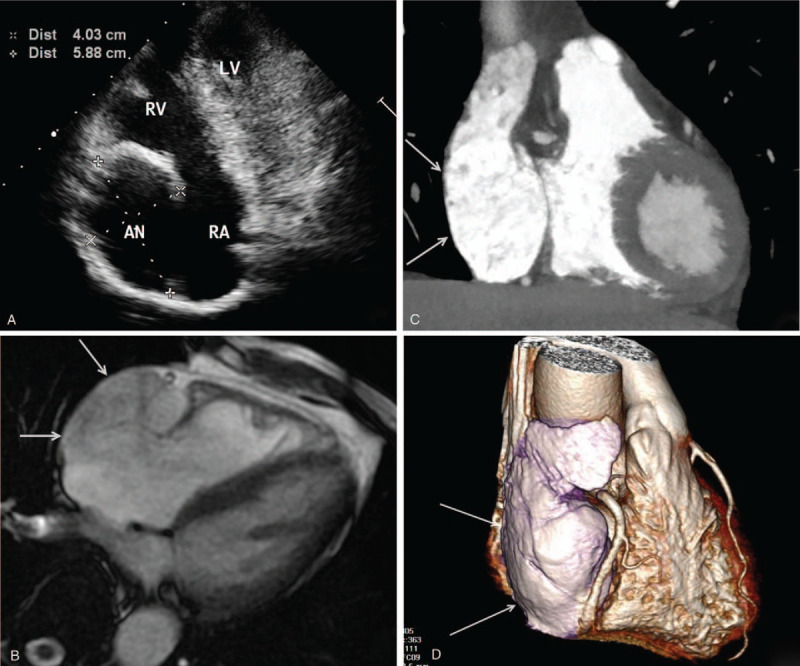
Ultrasonography, MR, and enhanced CT images obtained in case 1. (a) Ultrasonography revealed a cystic mass in the right edge of the heart, which was connected to the right atrium; (b) a diverticular lesion was seen inside the pericardium and found to be connected to the right atrium and to have a thin wall; (c and d) well-defined enhancement was observed in the localized extraneous lesion in the right atrium area, and a 3D image showed no external compressive structure in the adjacent coronary artery.
